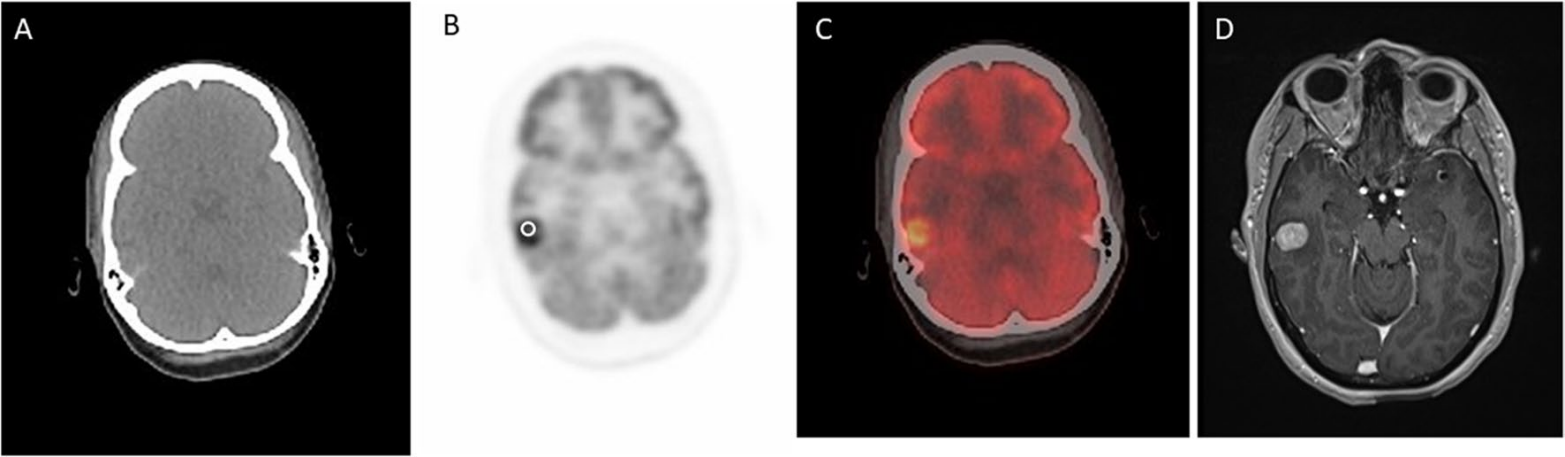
^18^F-FDG PET/CT and contrast-enhanced MRI images of a HER2-positive breast cancer metastasis. Non-contrast CT (A), ^18^F-FDG PET (B), fused ^18^F-FDG PET and CT (C), and axial T1-weighted post-contrast (D) images of a HER2-positive breast cancer brain metastasis in a 44-year-old woman. A representative region of interest is shown in white. This lesion had an SUVmax ratio of 4.99. ^18^F-FDG = ^18^F-fluorodeoxyglucose. SUVmax = maximum standardized uptake value
